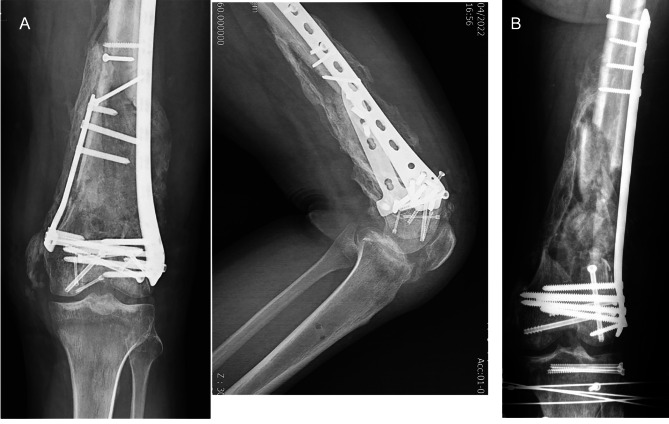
Follow-up X-ray after 3 months of bone grafting in a case with open C3 distal femur fracture. (A) Patient One (B) Patient Two
